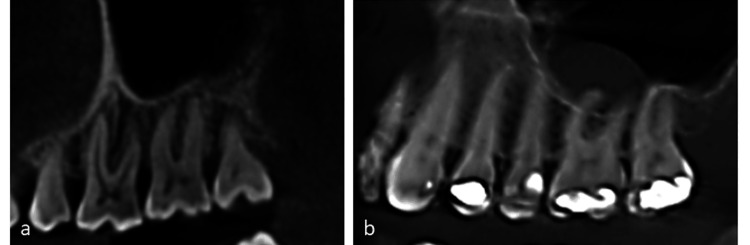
Cone-beam computed tomography sagittal sections a) no contact with root tips to the sinus cortical borders; b) the root tips are projecting into the sinus cavity.
